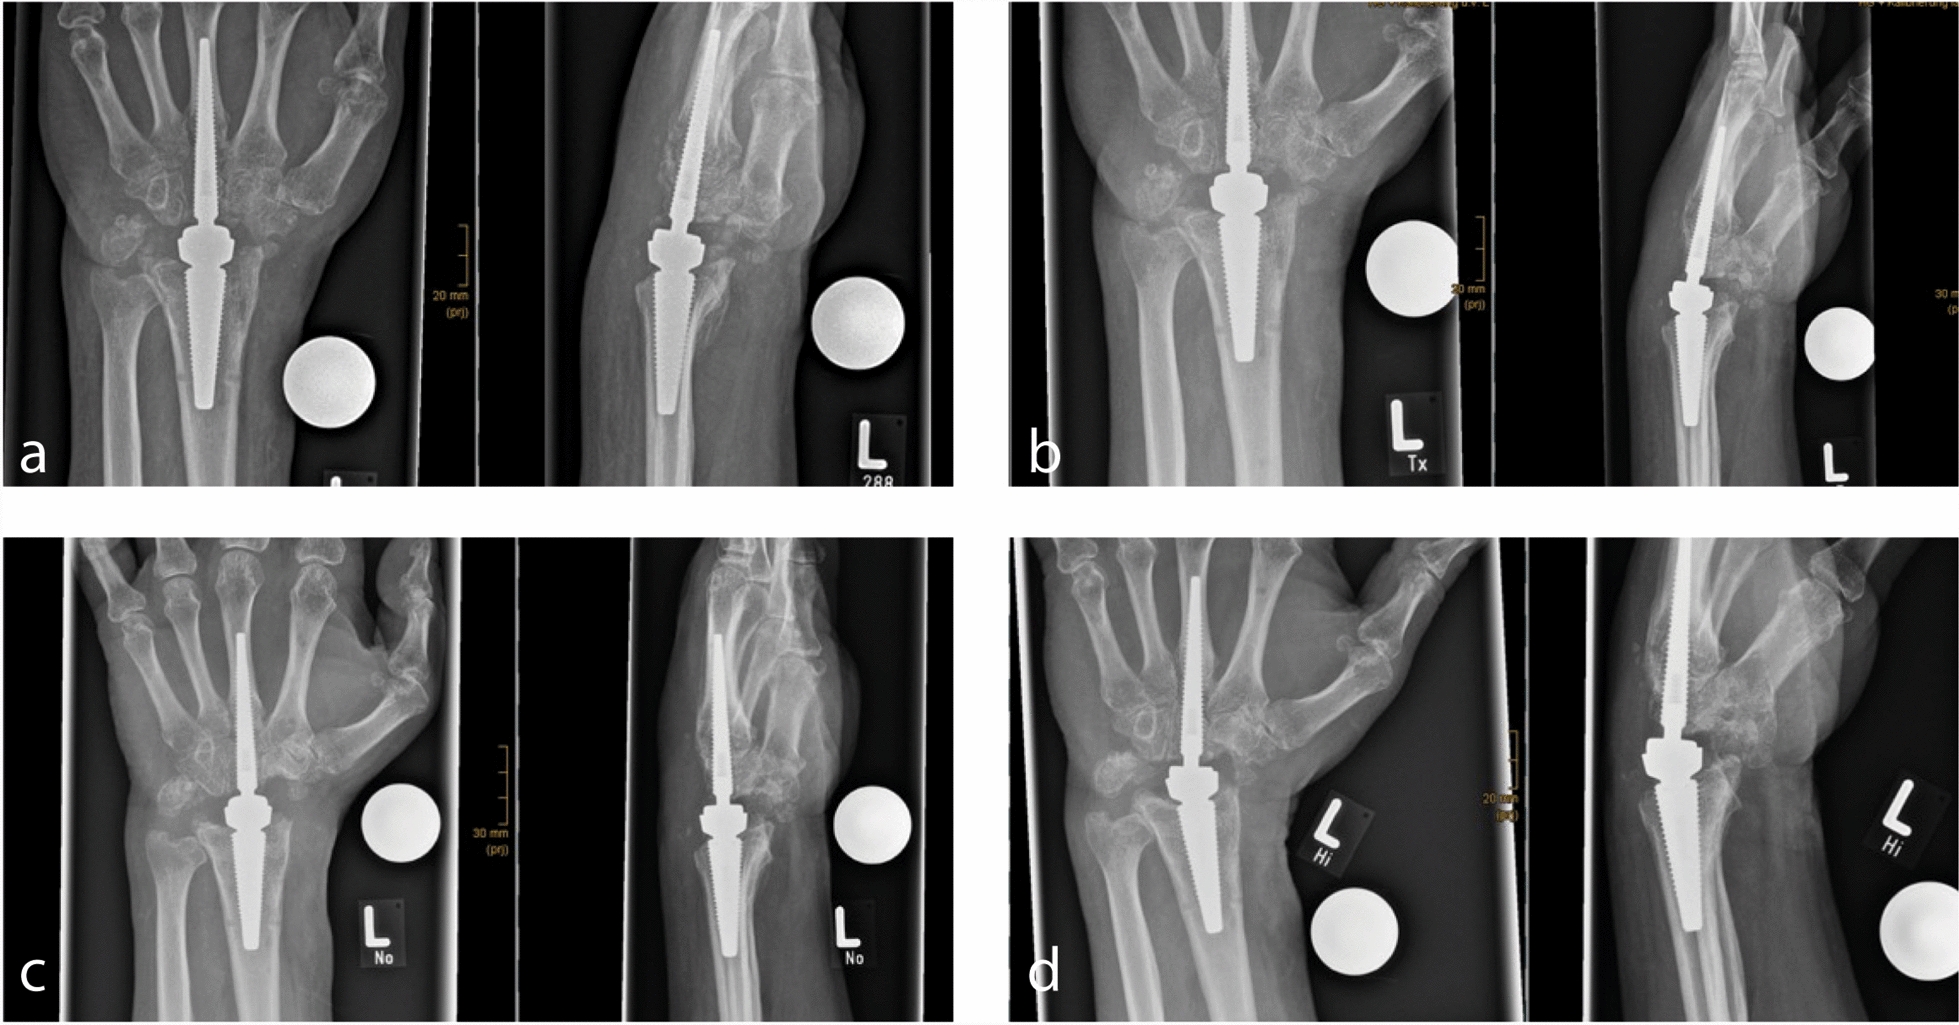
X-rays of the left hand as part of aftercare examination. a X-rays in anterior–posterior and lateral views, 6 weeks after revision surgery. b X-rays in anterior–posterior and lateral views, 3 month after revision surgery. a, c X-rays in anterior–posterior and lateral views, 6 months after revision surgery. d X-rays in anterior–posterior and lateral views, 1 year after revision surgery
